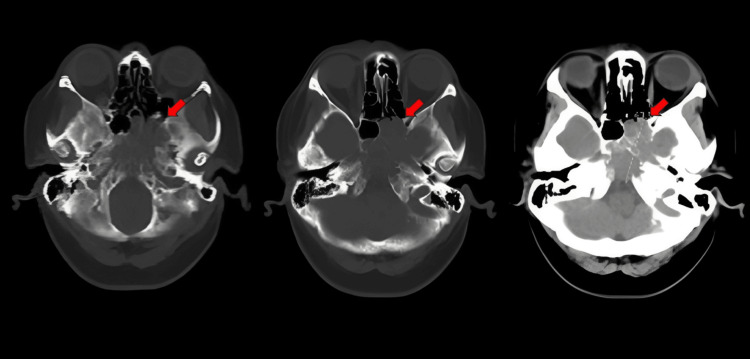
Preoperative non-contrast CT of the head showing a destructive clival mass (red arrows)
